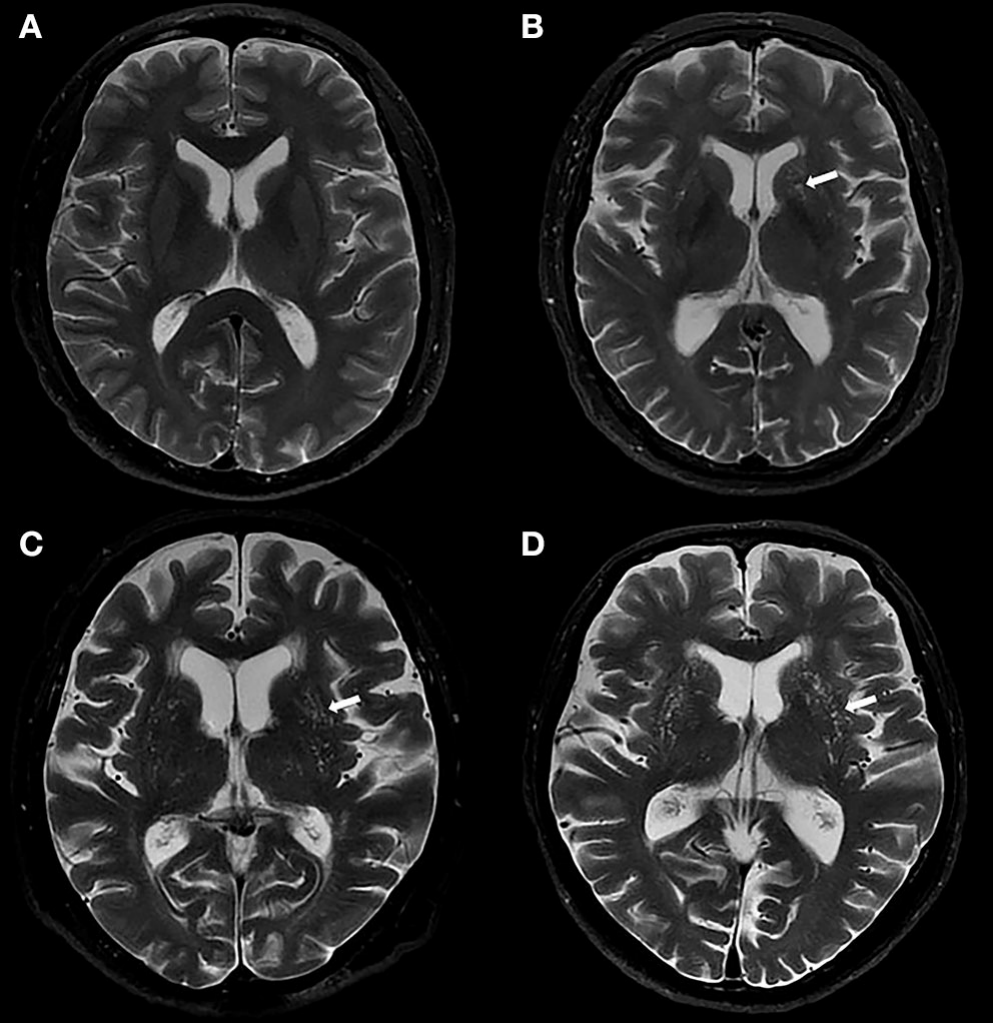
Examples of MRI-visible BG-PVS. (A,B) Axial T2-weighted images show the mild grade of BG-PVS (arrow), (C,D) Axial T2-weighted images show the severe grade of BG-PVS (arrow).
